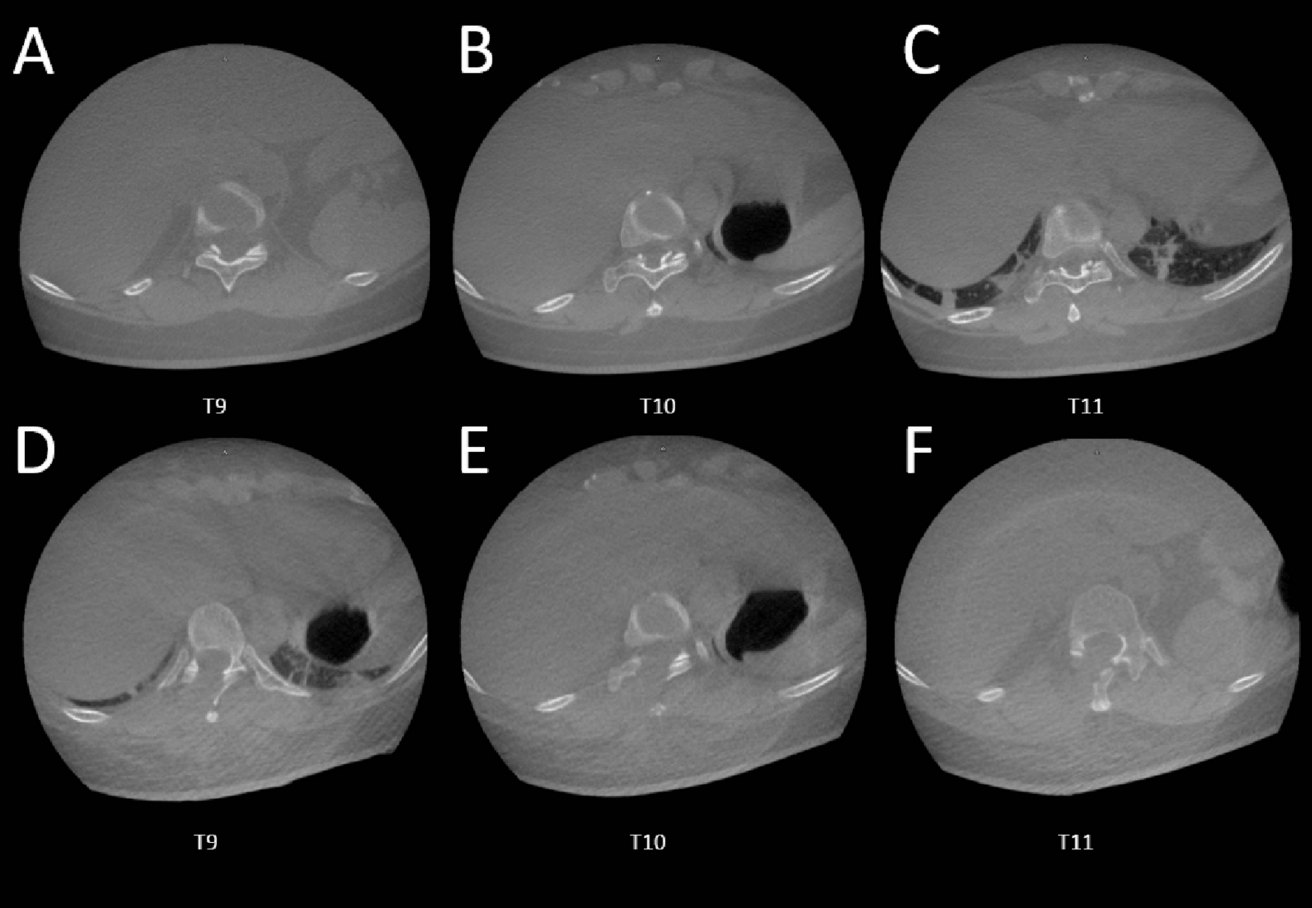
(A-C) Intraoperative CT scans demonstrating OYL at the T9, T10, and T11 levels. (D-F) Post-decompression intraoperative CT scans showing complete removal of the OYL. OYL: ossification of the yellow ligament
